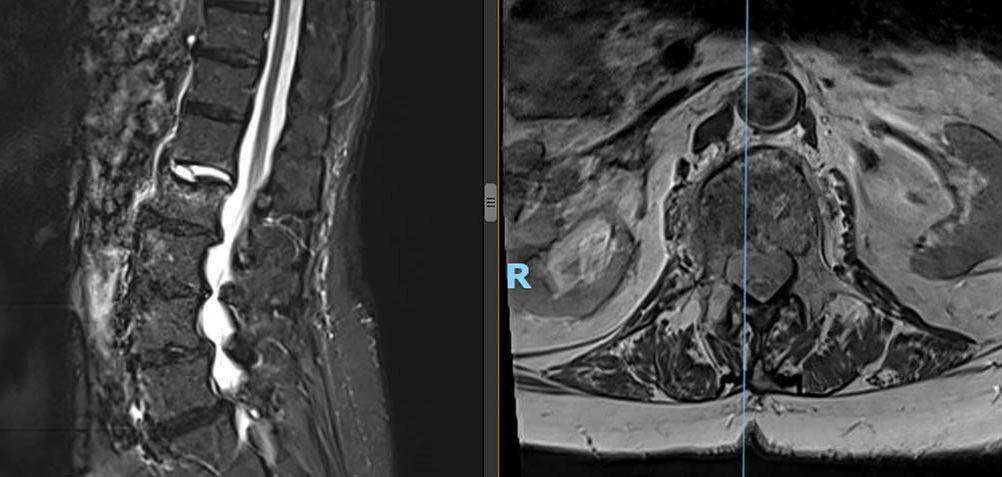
Preoperative lateral (left) and axial (right) MRI of the patient’s spine showing L2 compression fracture.
